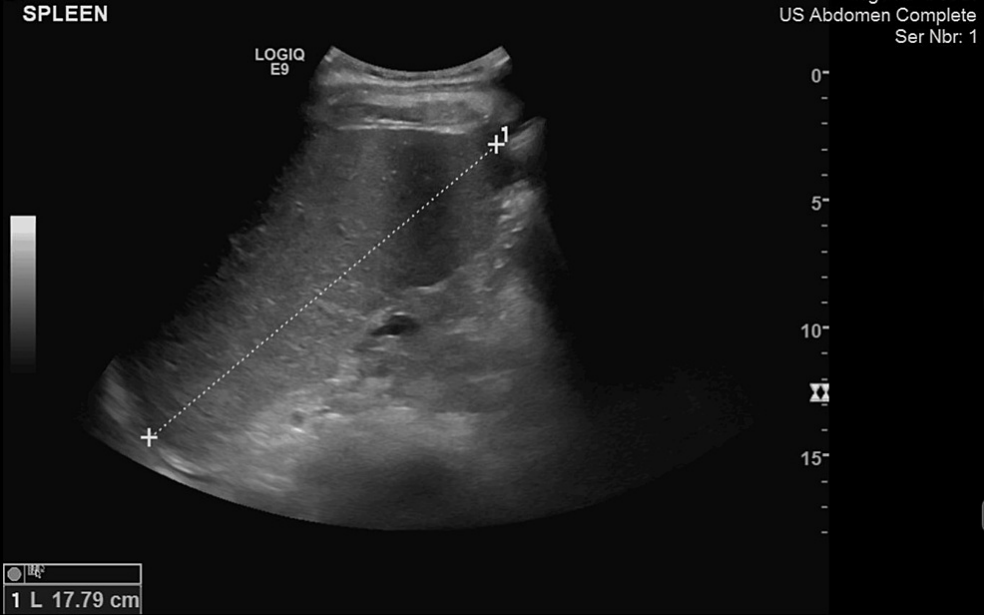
Ultrasound showing an enlarged spleen measuring 17.7 cm in length and normal homogenous echo-texture
